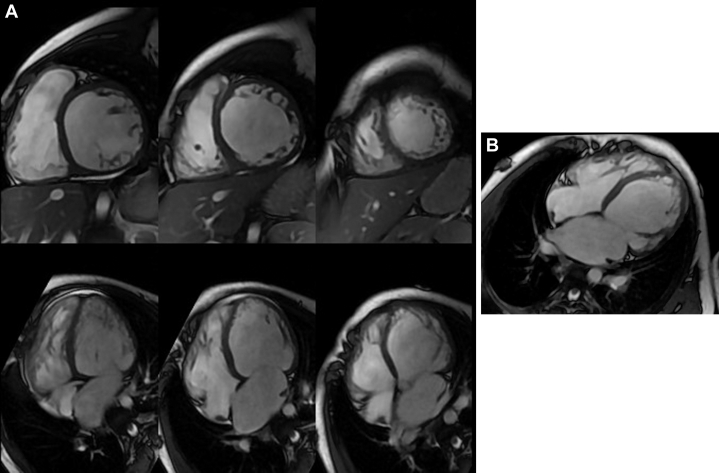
Cardiac Magnetic Resonance Image shows a dilatated and hypertrabeculated left ventricle with left ventricular ejection fraction of 23% and a dilated right ventricle with decreased right ventricular ejection fraction (27%). (B) Image shows a small area of delayed enhancement at the level of the anterior septum.
